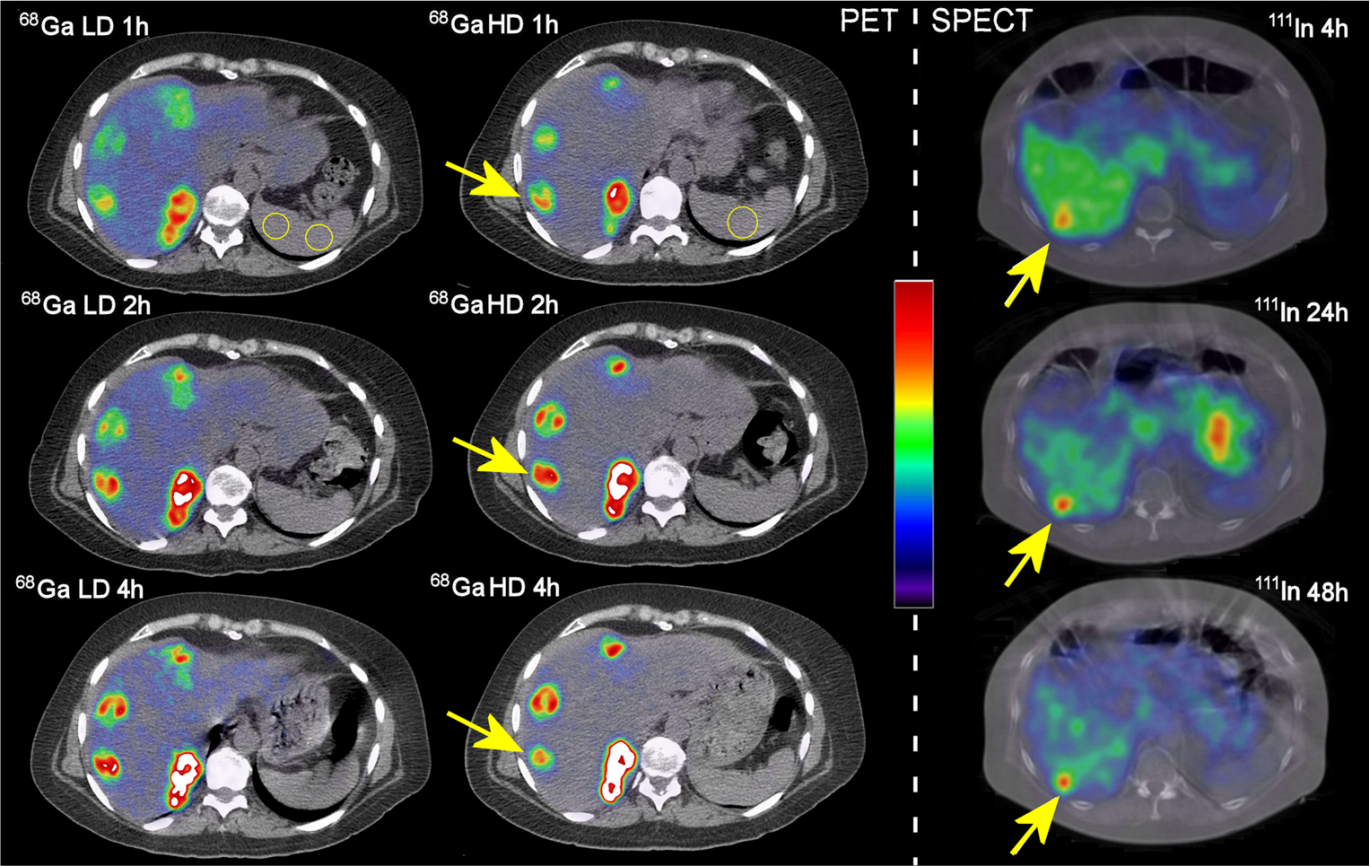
Examples of abdominal PET/CT and SPECT/CT images including normal liver, liver metastases and spleen for reference. Left column: low peptide dose [^68^Ga]-ABY-025-PET/CT at 1, 2, and 4 hours after injection. Middle column: high peptide dose [^68^Ga]-ABY-025-PET/CT at corresponding time points in the same patient. Right column (other patient): [^111^In]-ABY-025--SPECT/CT at 4, 24, and 48 hours after injection. The PET colour scale is thresholded at SUV 32. The SPECT images are corrected for the decay of ^111^In to represent uptake and the colour scale arbitrarily normalised. Arrows indicate a liver metastasis of breast cancer in each of the two patients. Examples of dual spleen VOI:s (spherical) and single slice circular ROI are shown in yellow (first row PET images). ^68^Ga LD and ^68^Ga HD stand respectively for low and high peptide content [^68^Ga]-ABY-025. ^111^In stands for [^111^In]-ABY-025
